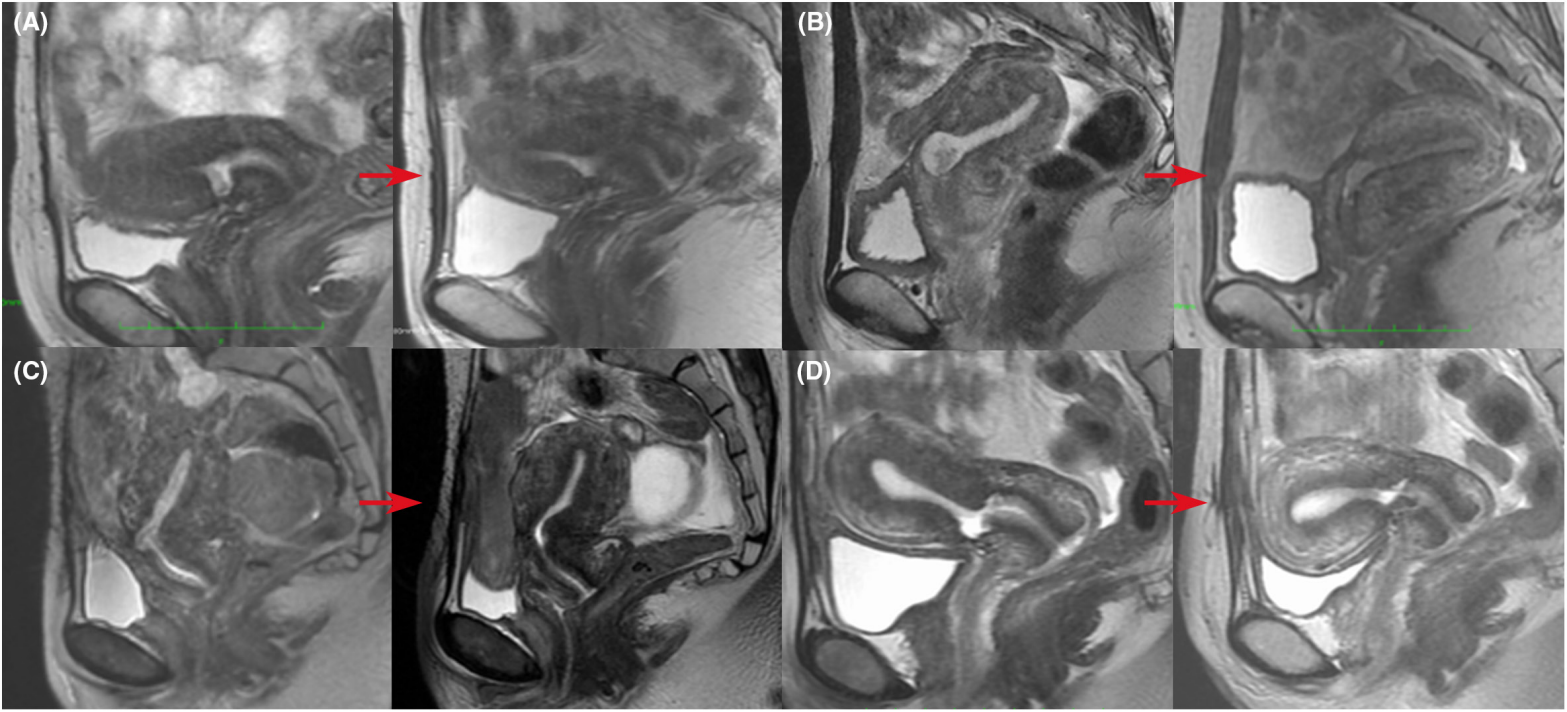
Magnetic resonance imaging findings before and after hysteroscopic surgery. Hysteroscopic surgery was performed for infertile women with cesarean scar disorder (CSDi) in each of the four independent cases (A–D). In all cases, the residual myometrial thickness increased, and postoperative pregnancies were established in all cases. Red arrows indicate pre‐ and post‐operative changes.
